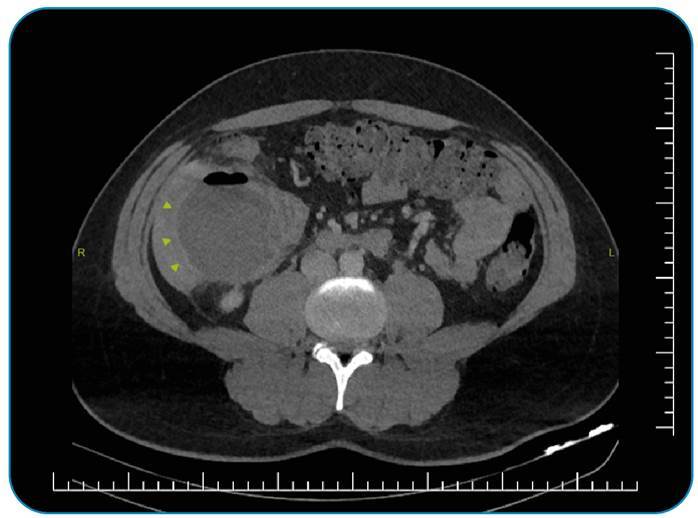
CT shows right hepatic lobe subcapsular abscess (arrowheads).
